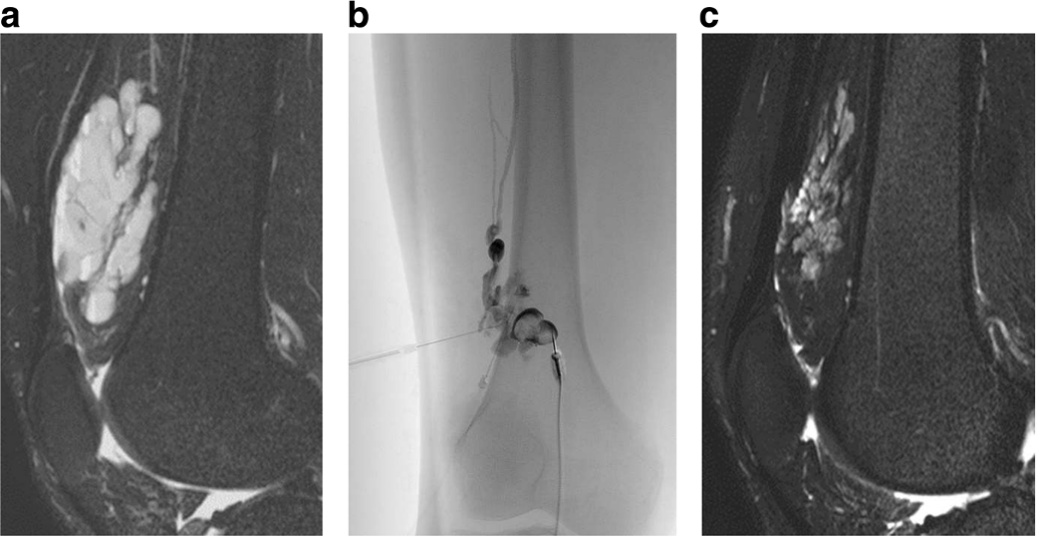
A 30-year-old-male with pain, swelling, and dysfunction of the right knee joint. A. The sagittal fat-saturated T2-weighted MR image before treatment shows a lobulated high-intensity mass in the suprapatellar bursa. B. Direct puncture phlebography shows the lesion cavity and the conducting vein (Type 2). Sclerotherapy was performed with 3% polidocanol foam. C. After two sessions, MR imaging shows a decrease in size and signal intensity of the mass. The patient had improvement of symptoms and indicated satisfaction on the questionnaire.
